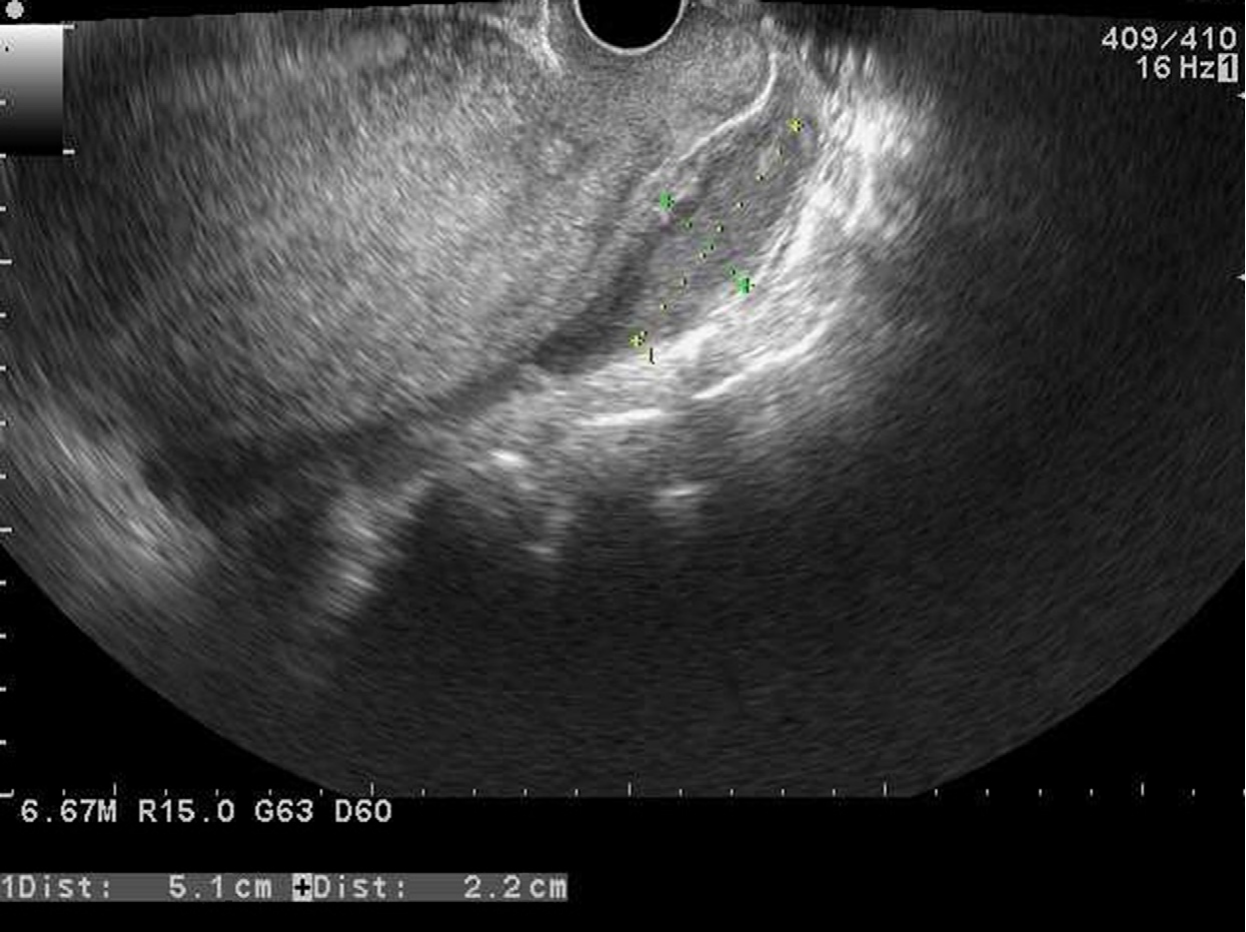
Transvaginal ultrasound image taken 3 days postpartum confirming rupture of the ovarian endometrial cyst in Douglas' pouch.
